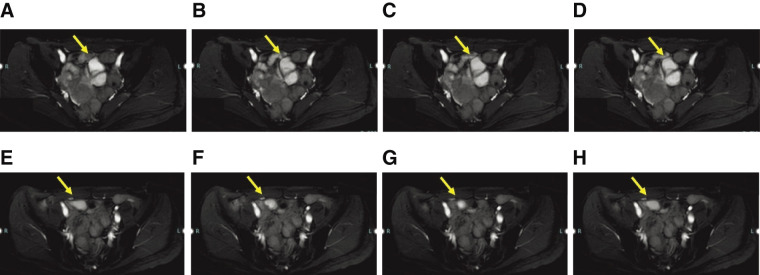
Pelvic magnetic resonance imaging showed a cystic lesion on the left side of the pelvis (arrows A–D). On cine imaging, the cyst appeared to be peristaltic (A, after 0 s; B, after 10 s; C, after 20 s; and D, after 30 s). Pelvic magnetic resonance imaging 1 month later showed a cyst-like structure in the right pelvis, changed from the previous one (arrows E–H). As before, peristalsis was observed by cine imaging (E, after 0 s; F, after 10 s; G, after 20 s; and H, after 30 s)
